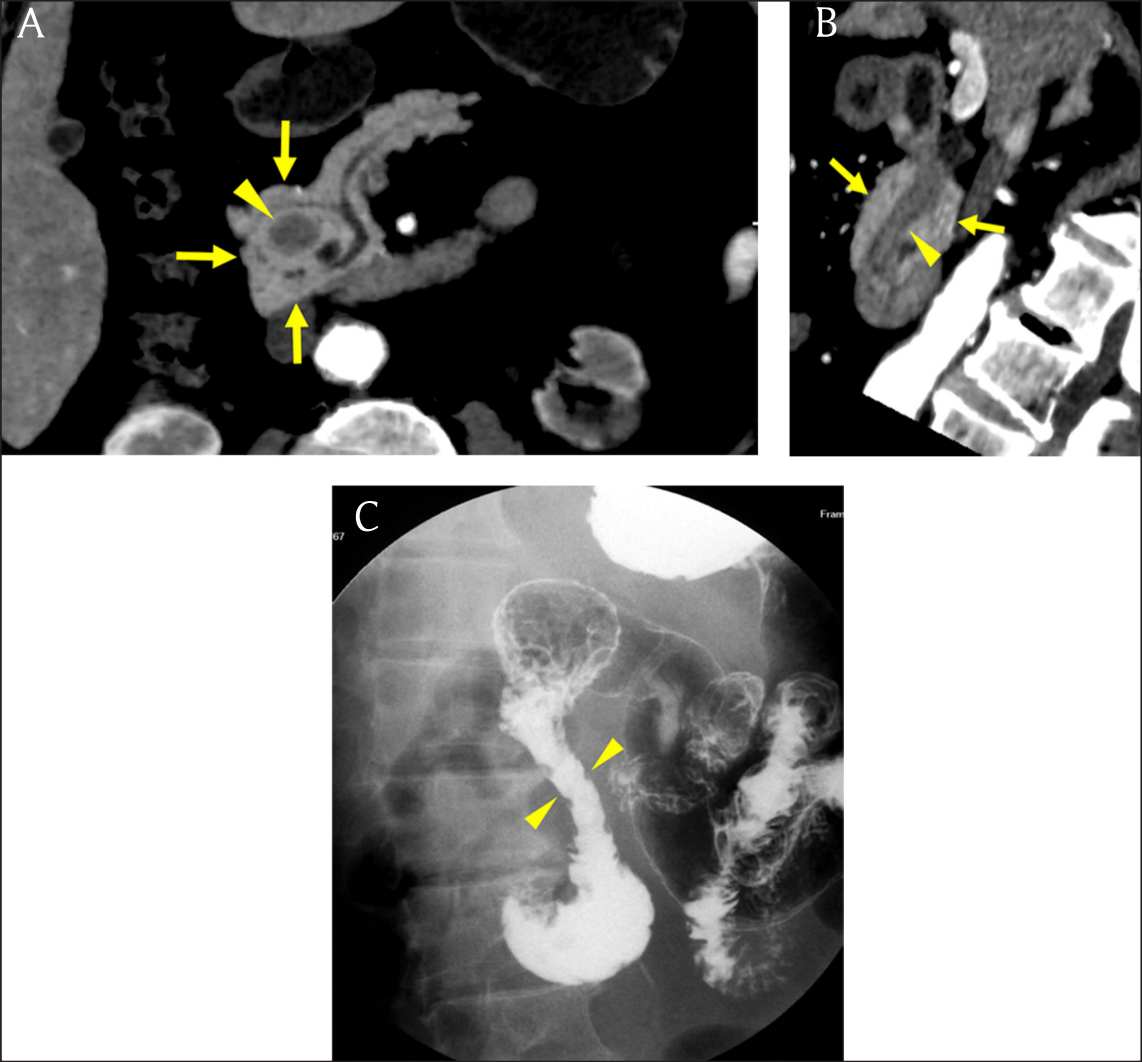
A 67-year-old man with annular pancreas. (A, B) Curved reformatted pancreatic phase CT images show pancreatic tissue (arrows) and duct around the second part of the duodenum (arrowhead). (C) Upper gastrointestinal series image shows concentric luminal narrowing of the descending duodenum (arrowheads).
